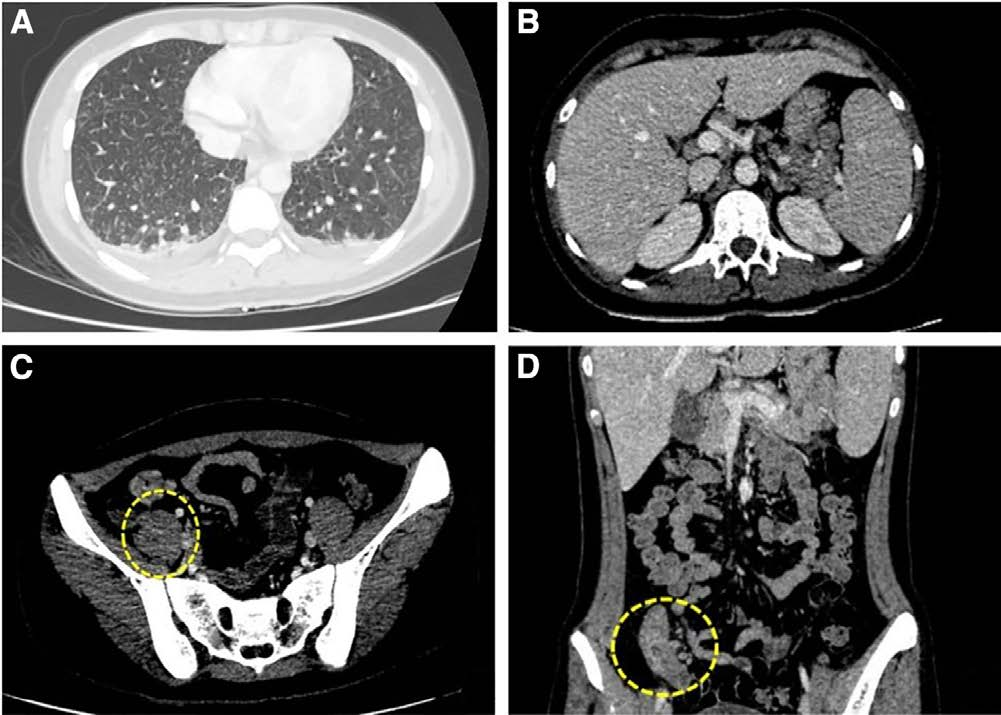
Imaging findings in a 20-year-old female with LE. (A) Chest CT showing reticular patterns and subpleural interlobular septal thickening, indicative of lupus-associated interstitial lung changes similar to UIP, without cavitary lesions. (B–D) Contrast-enhanced abdominal CT demonstrating diffuse non-necrotic lymphadenopathy (red arrows, B), inflammation of the terminal ileum with circumferential and symmetrical mural thickening of the terminal ileum with mild surrounding fat stranding (yellow circles, C), and partial narrowing at the ileocecal valve (yellow dashed circle, D). CT = computed tomography, LE = lupus enteritis, UIP = usual interstitial pneumonia.
